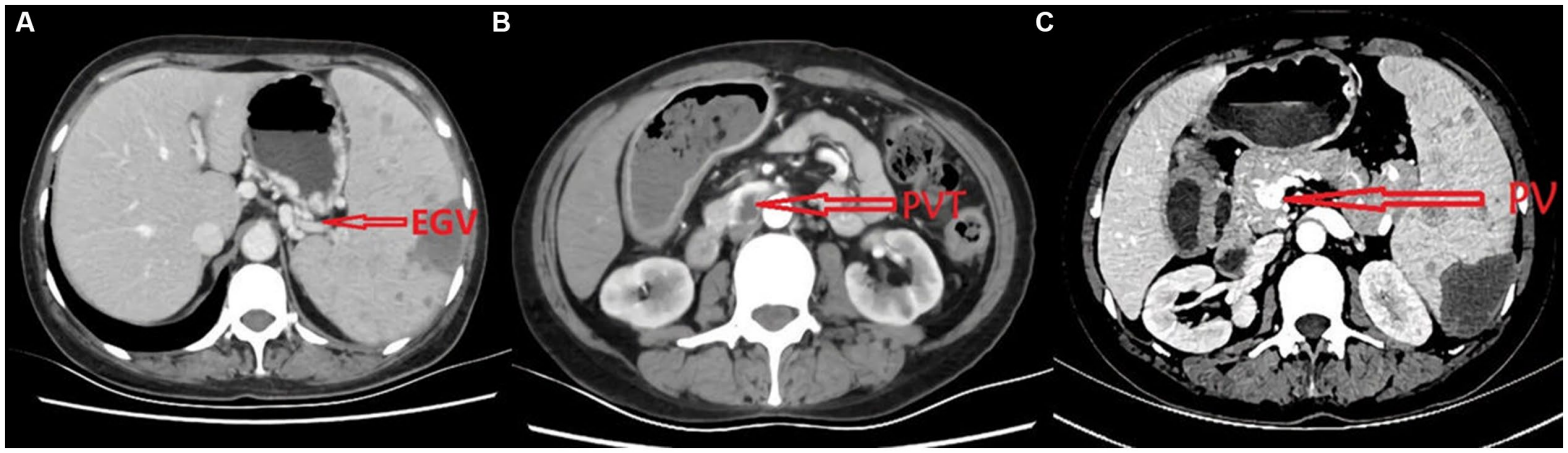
The CT scan before SSAE (A), before TIPS (B), and after TIPS (C).
